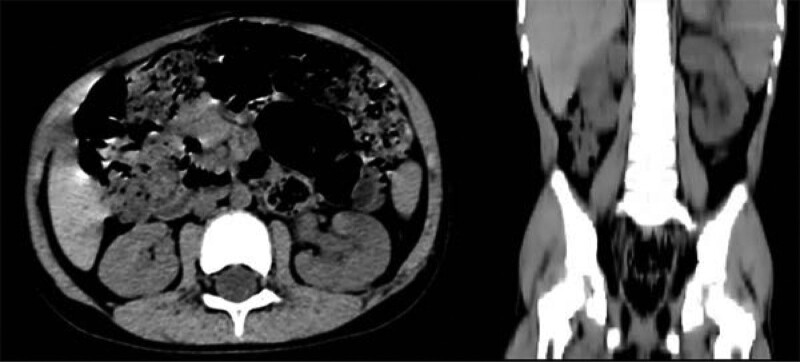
Sagittal and coronal view of postoperative lower abdominal CT (Case 2). CT = computed tomography.
